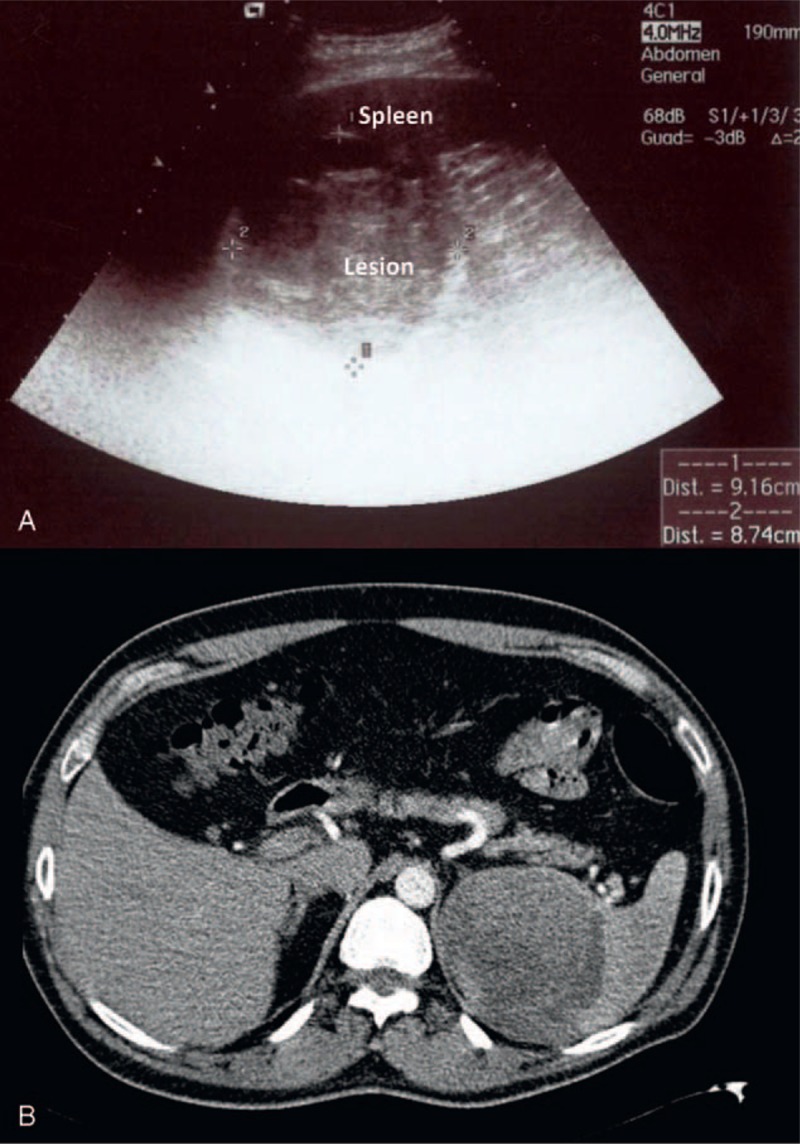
(A) Ultrasound of the spleen showing a large 9 × 8 cm solid lesion at the upper pole, with mixed echogenicity, prevalently hyperechoic, and almost no vascularization; (B) Arterial phase helical CT scan showing a 76 × 76 mm round mass, with dishomogeneous hypodense and high-density areas located at the upper pole of the spleen, with a thick subcapsular fluid collection at the inferior pole (not visible in this section).
